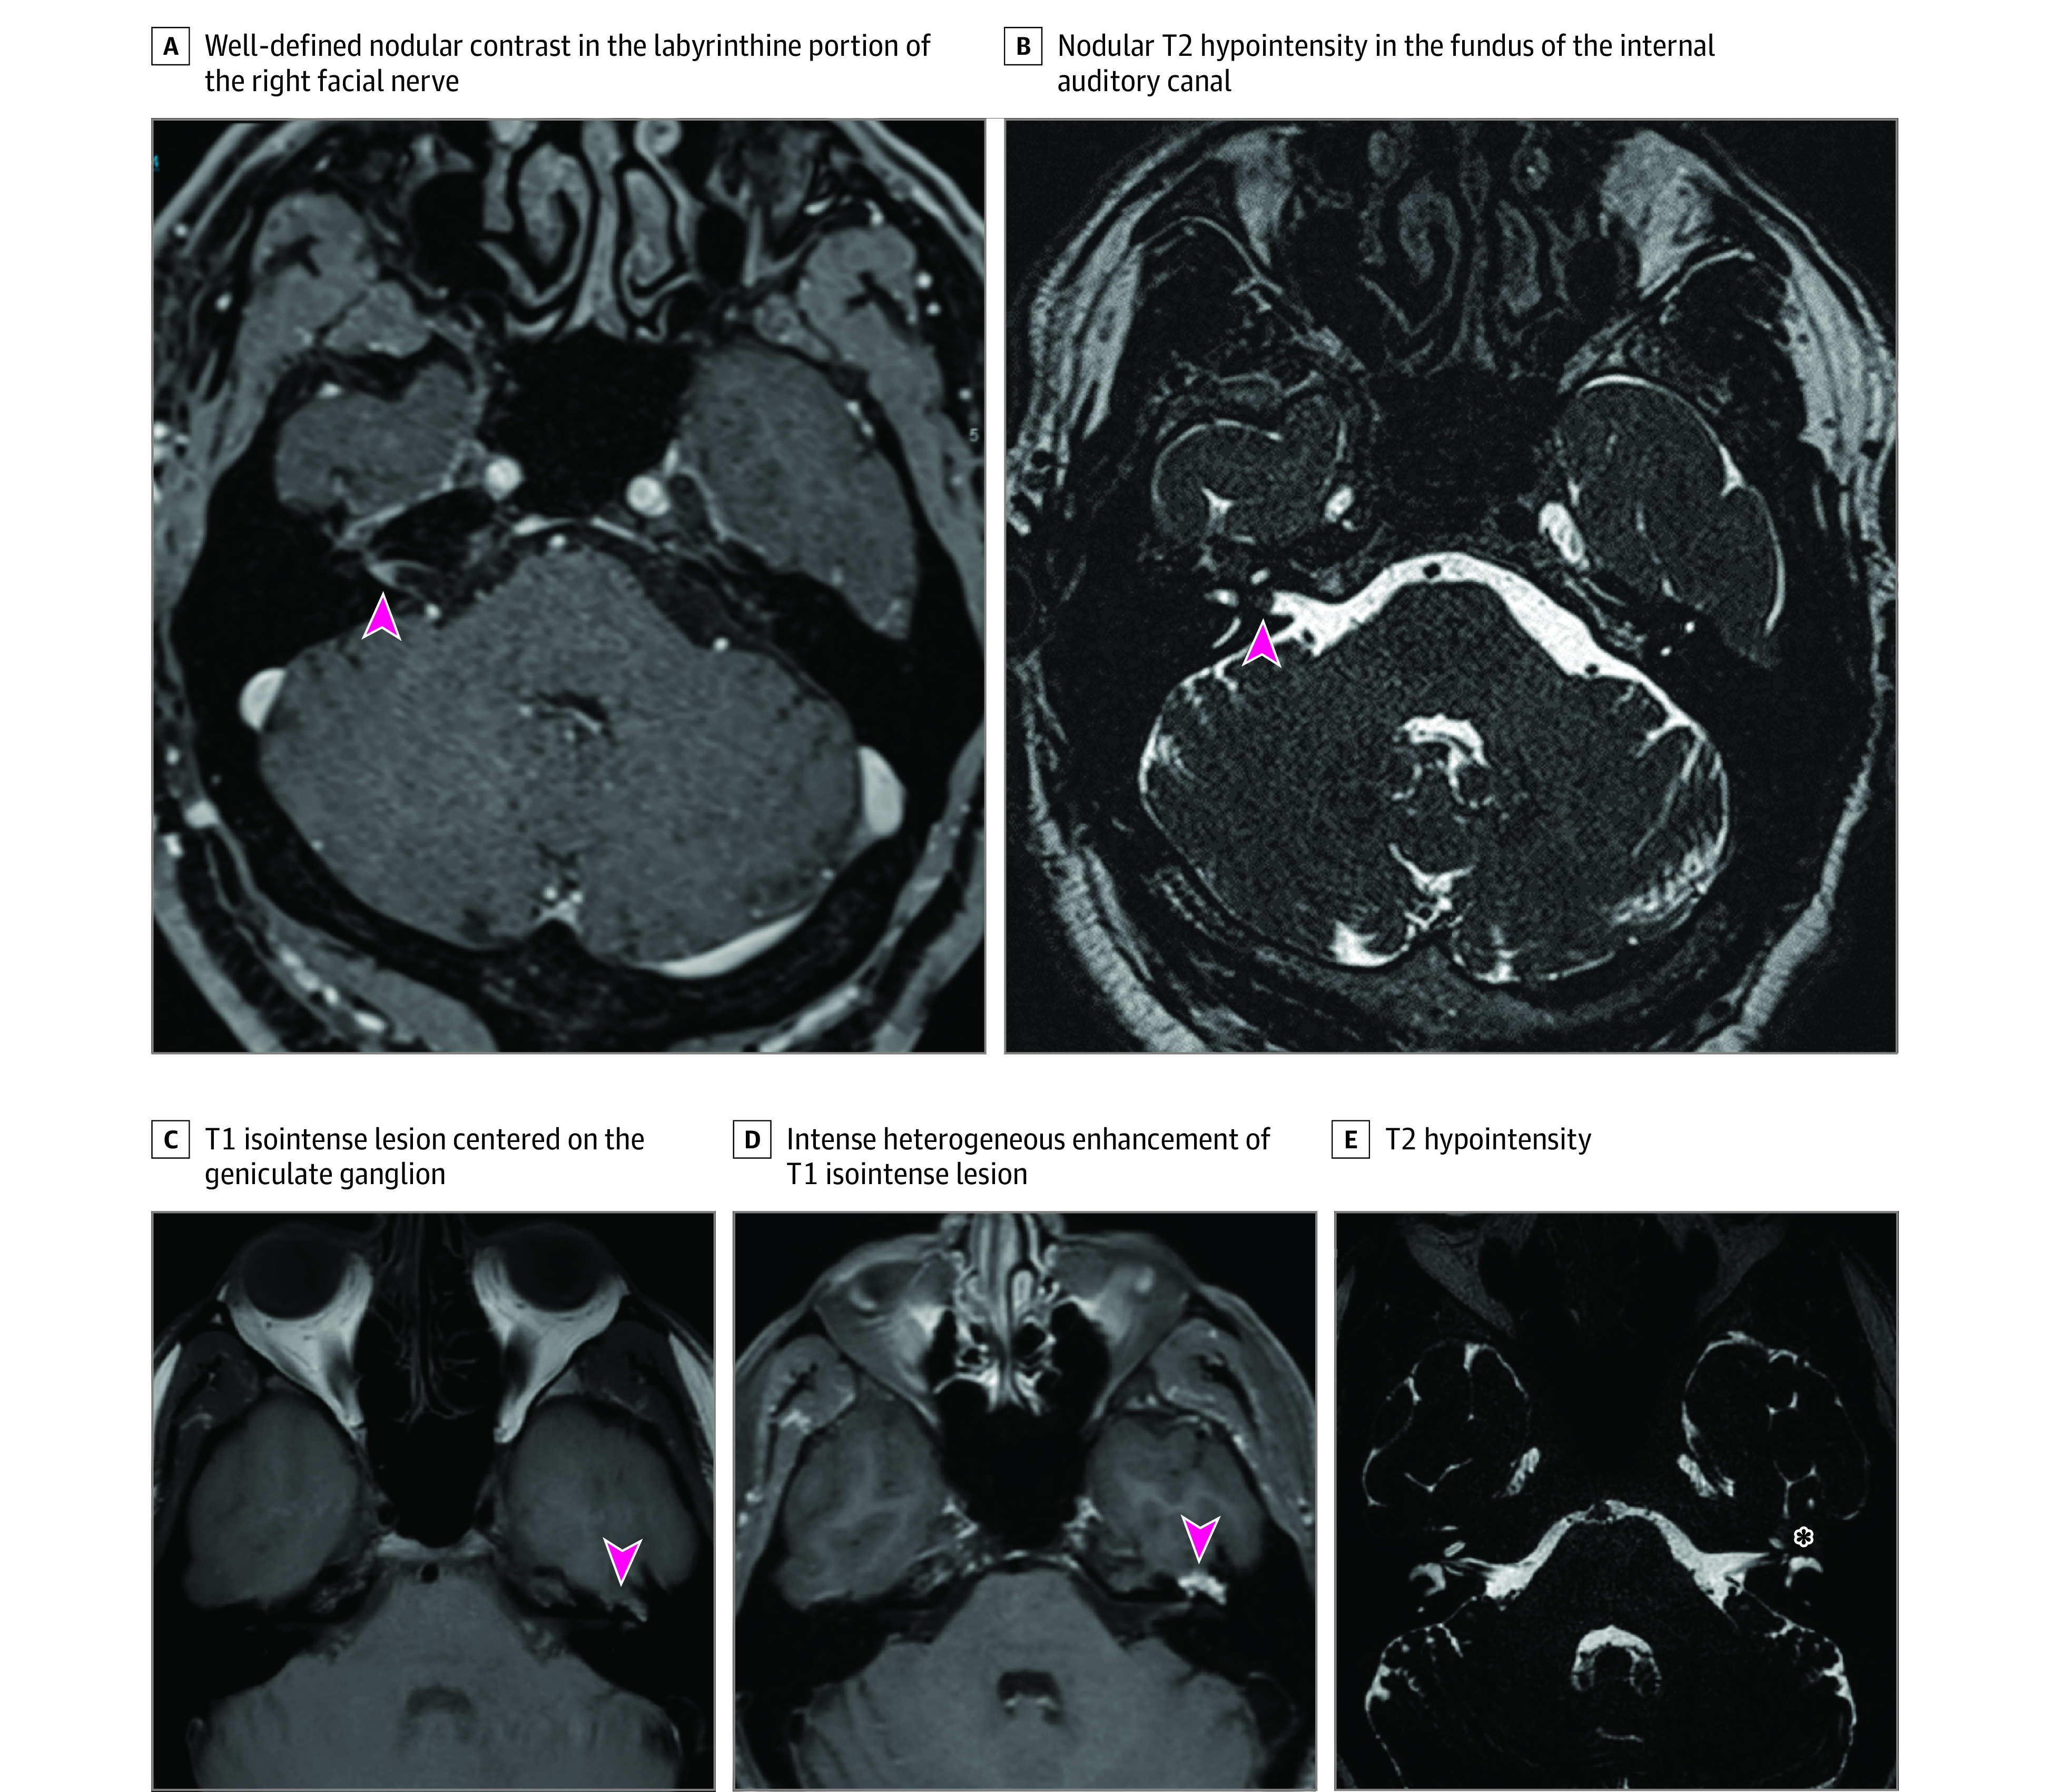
Magnetic Resonance Imaging of Patients With Peripheral Facial Palsy (PFP) Due to Facial Nerve Schwannoma and Facial Nerve Hemangioma A, Contrast-enhanced T1-weighted image in a patient in their 50s with right PFP due to facial nerve schwannoma showing well-defined nodular contrast in the labyrinthine portion of the right facial nerve (arrowhead). B, Nodular T2 hypointensity in the fundus of the internal auditory canal (arrowhead) in the same patient. C, T1 isointense lesion centered on the lateral part of the geniculate ganglion (arrowhead) in a patient in their 40s with left PFP due to facial nerve hemangioma. D, Intense heterogeneous enhancement after gadolinium injection (arrowhead) in the same patient. E, T2 hypointensity (asterisk) in the same patient.
